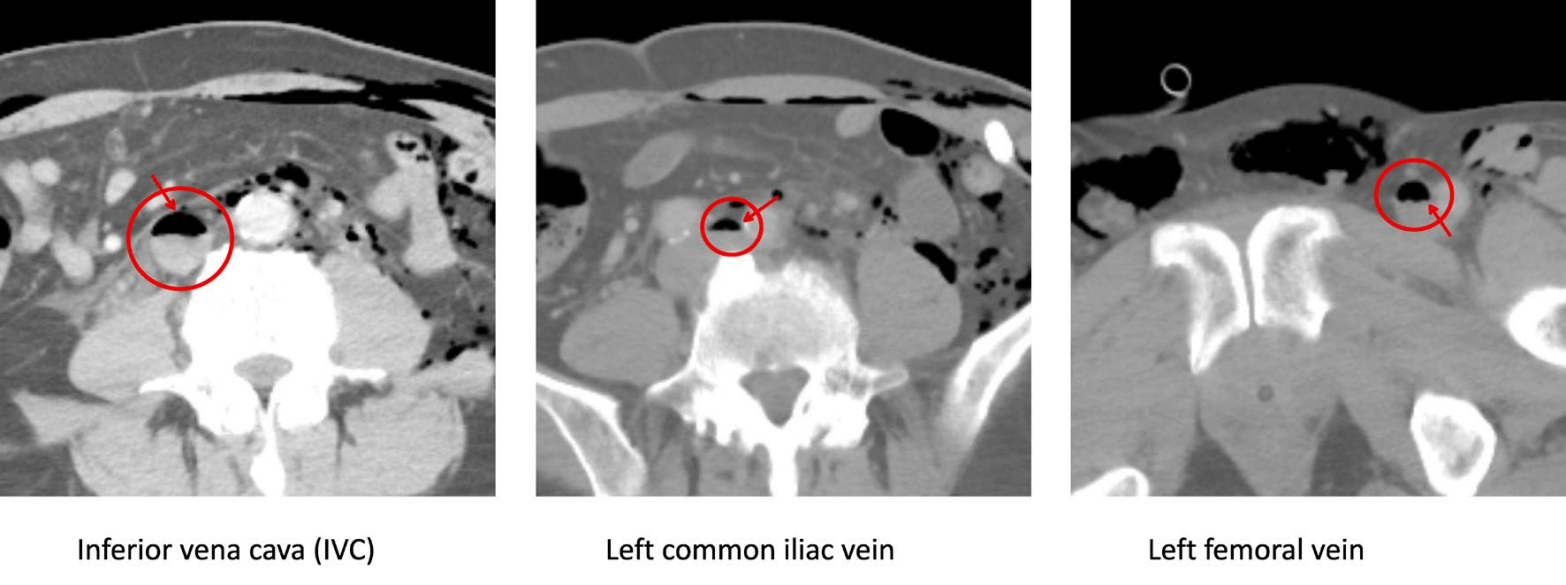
Postoperative CT findings. A mirror image of CO_2_ extending from the inferior vena cava (IVC) to the left femoral vein was observed.
